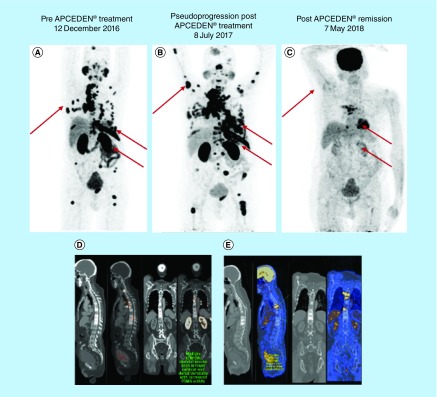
PET-CT scan of patient before APCEDEN treatment and after successfully completing six doses of the dendritic cell immunotherapy regimen. (A–C) Full body PET scan images comparing the patient’s metastasis, pseudoprogression (2 months after last dose of APCEDEN) and substantial remission of metastatic lesions (12 months after last dose of APCEDEN), respectively. Changes in tumor size is indicated by red arrows in the image. (D & E) PET scan image of vertebrae exhibiting decrease in metastatic lesions post-APCEDEN therapy.
